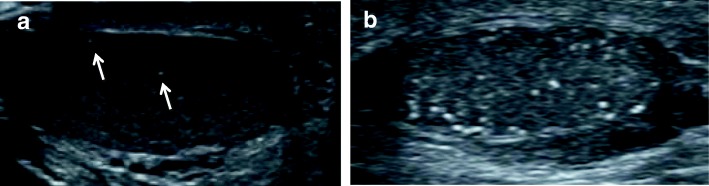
Representative pictures of LTM and CTM. a Limited testicular microlithiasis (arrows) in an 11-year-old boy with hypospadias and undescended testis. Ultrasonography of right testis showed 2 small, uniform-appearing echogenic foci without acoustic shadowing. His left testis also displayed 4 echogenic foci per field. b Classic testicular microlithiasis in a 1-year-old boy with hypospadias. Ultrasonography of left testis demonstrated more than 5 echogenic foci per field. Right testis also displayed more than 5 echogenic foci per field
